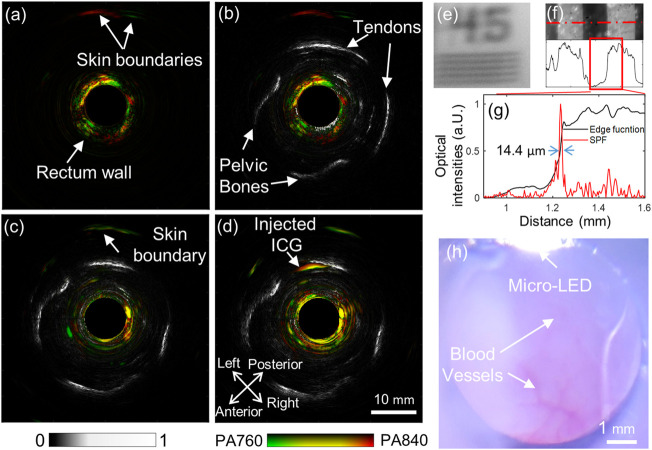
Multi-functional endoscopic imaging results of the rabbit rectum. (A) Fused photoacoustic image of 760 and 840 nm; (B) Fused dual-wavelength photoacoustic and ultrasound image; (C) and (D), fused dual-wavelength photoacoustic and ultrasound images before and after a dose injection of ICG, respectively; (E) and (F) are the acquired wide-field optical endoscopic image of a positive resolution test target plate, with patterns of 45 circle/mm and 1.0 circle/mm, respectively; (G) Edge function (black) and derived PSF (red) of the wide-field optical endoscopy, obtained from the data along the dotted red line in (F); (H) The wide-field optical image of the rectum inner surface.
